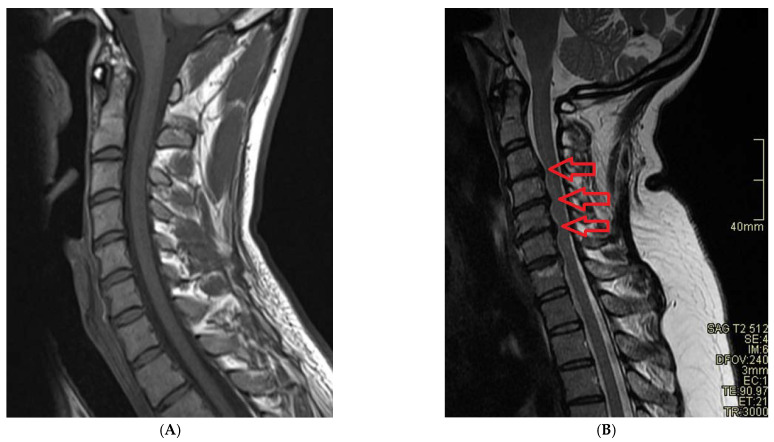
(A) Normal sagittal cervical spine T1 MRI; (B) 50 year old female patient with straightened cervical curvature is noted due to muscle spasm, in addition to advanced spondylodegenerative changes and multiple disc bulges (red arrows) of the cervical vertebrae. Reduced hydration of all cervical discs and sub end plate marrow degenerative changes in C3–C4, C4–C5 are also observed.
